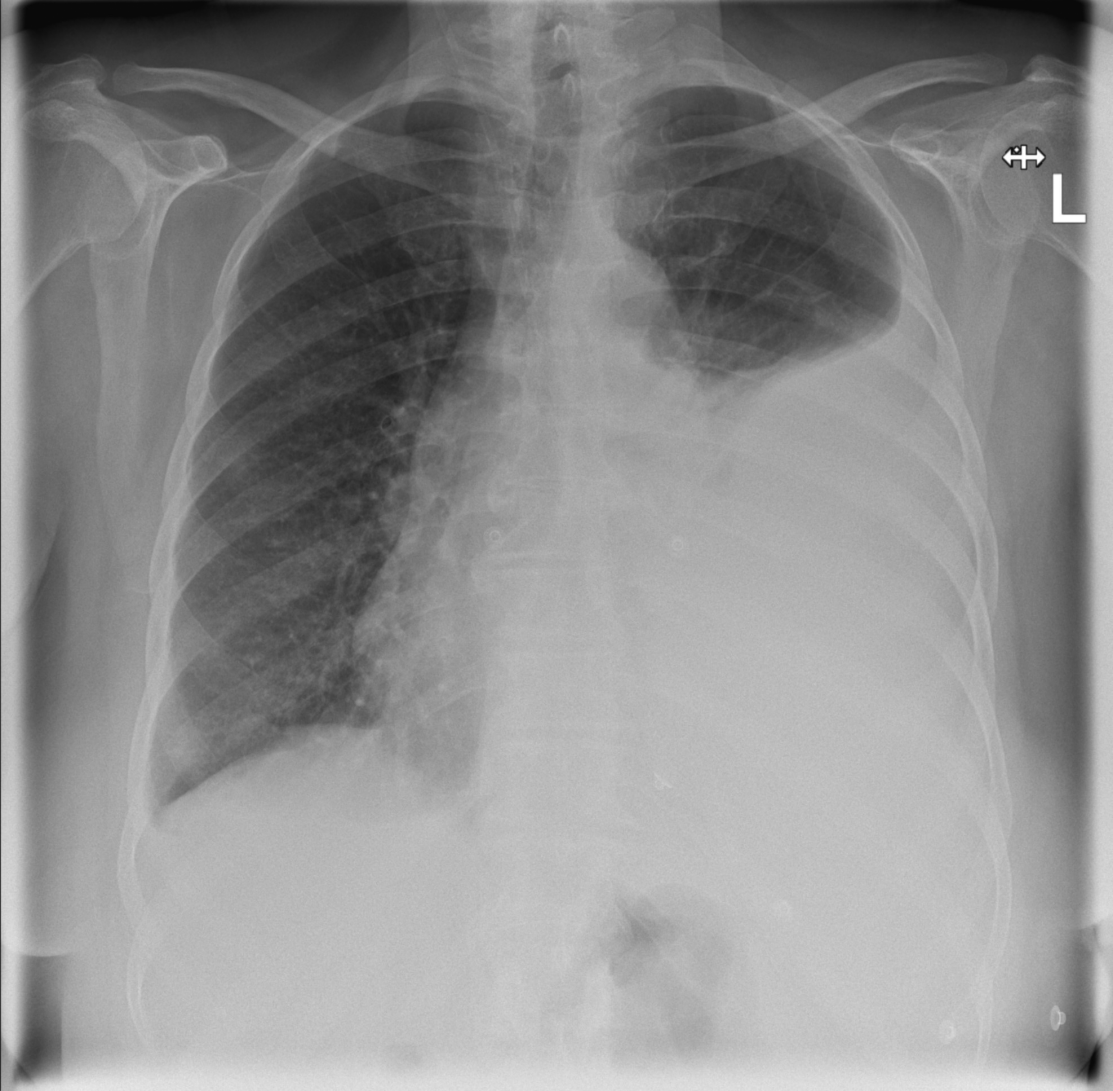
Initial chest x-ray with left pleural effusion.
